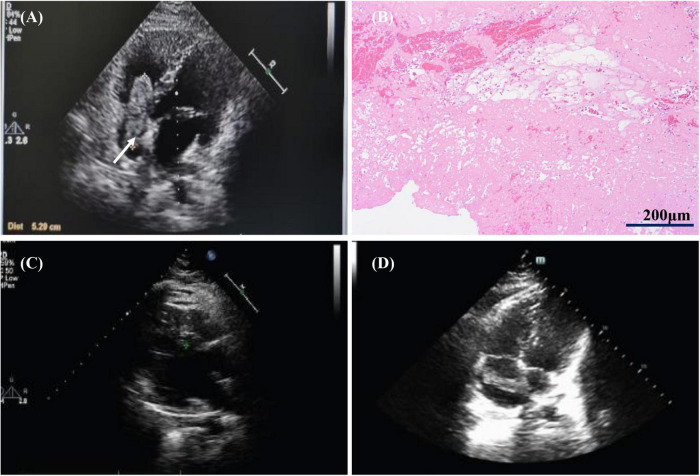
Images of first hospitalization and recurrence of right atrial mass. (A) The first echocardiography revealed a right atrial mass. (B) Pathological image of right atrial mass: a small number of platelets and inflammatory exudates. (C) Echocardiography at 10 days after operation showed that the right atrial mass disappeared. (D) Right atrial mass revisited (Less than one month after surgery) White arrows represent thrombus.
